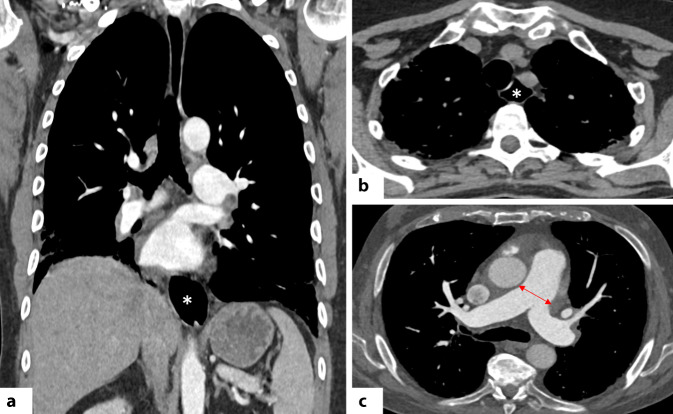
Koronale und axiale CT-Aufnahmen bei Patientinnen mit systemischer Sklerose zeigen eine Ösophagusdilatation (*weiße Sternchen*) im distalen (a) bzw. oberen Mediastinum (b). Axiale kontrastverstärkte CT (c) bei einer Patientin mit SSc mit pulmonaler Hypertonie (PH): deutliche Erweiterung des Truncus pulmonalis (*roter Pfeil*). Ein Trunkusdurchmesser >29 mm sowie ein Verhältnis Durchmesser zu Pulmonalarterie/aufsteigender Aorta (PA/A) > 1 gelten als hinweisend auf eine PH
